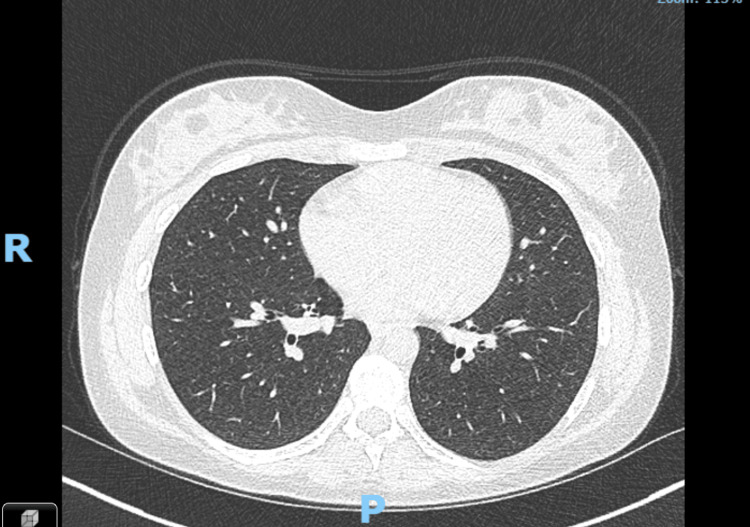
Repeat high-resolution CT of the thorax: there is complete resolution of previously noted extensive ill-defined centrilobular nodularity with significant improvement in the mosaic attenuation pattern. There are persistent areas of subtle low density in the lower lobes corresponding to previous areas of air trapping. No concerning focal lung lesion or consolidation CT: computed tomography
